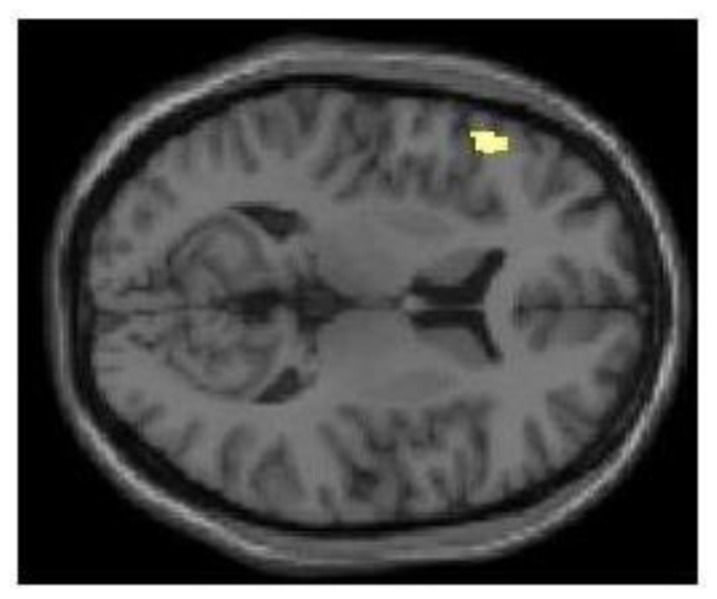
Following sleep deprivation the left inferior frontal gyrus was activated more when generating uses (fluency) in the divergent thinking task. Following sleep deprivation, there was greater activation in left inferior frontal gyrus when generating uses (compared to recalling characteristics) in the Alternate Uses Task. SPM rendered into standard stereotactic space and superimposed on to transverse MRI in standard space.
